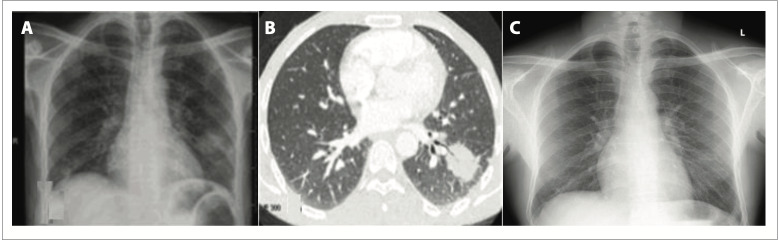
Initial chest X-ray imaging showing left lower lobe mass with smaller pulmonary nodules (A); contrasted chest computed tomography showing
